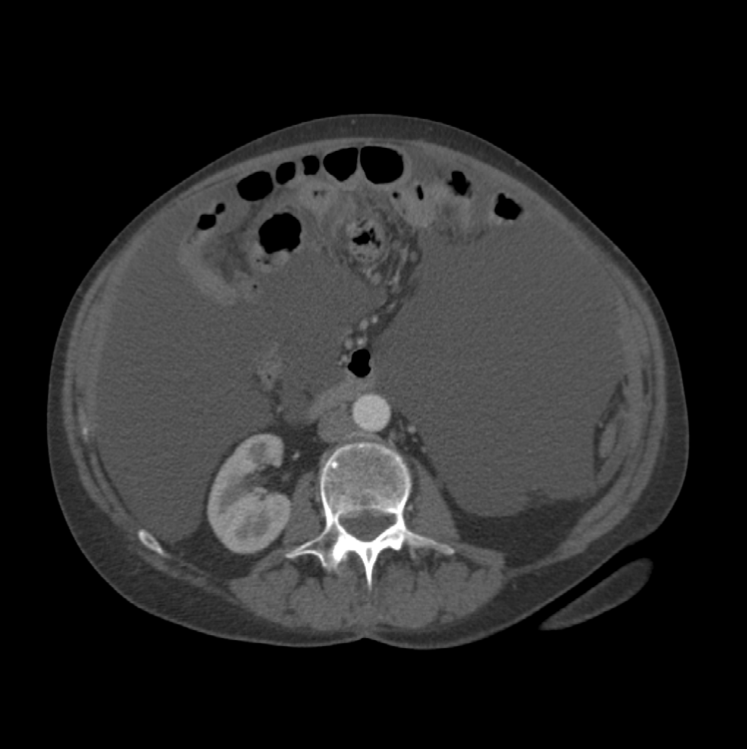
CT scan of the abdomen showing omental nodularity.
